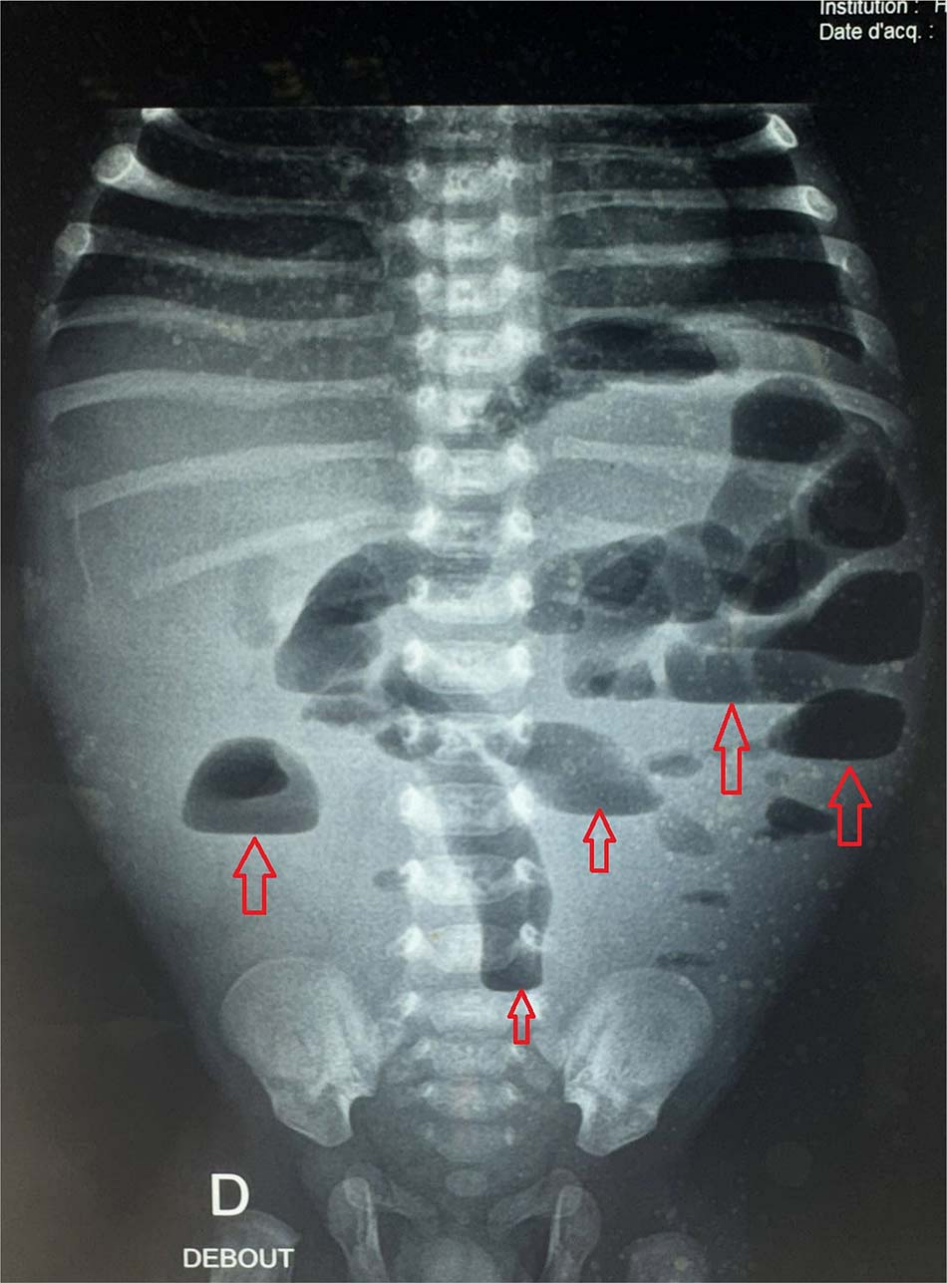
Postoperative abdominal radiograph demonstrating multiple air-fluid levels (arrows) without evidence of significant colonic dilatation.
